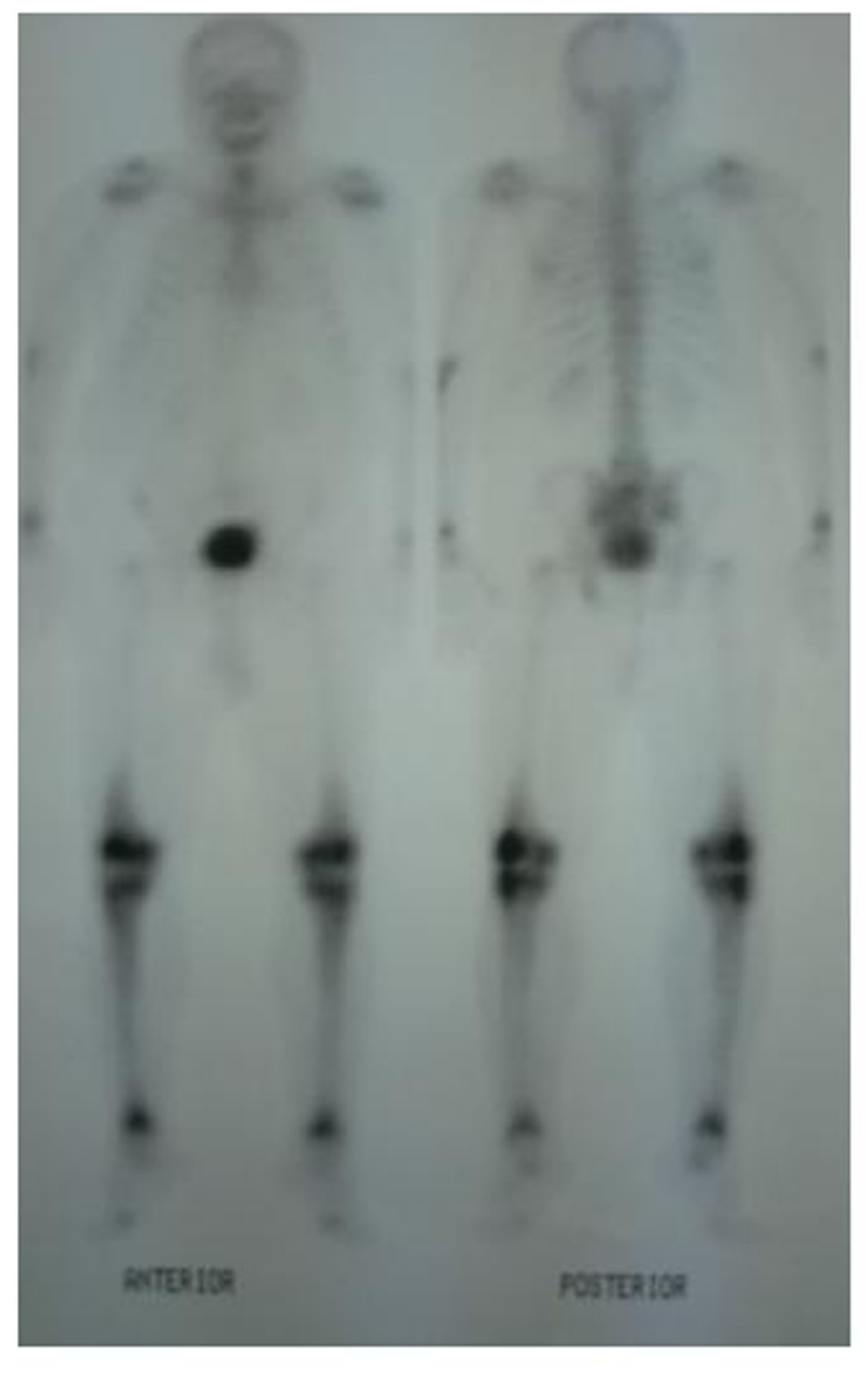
Increased osteoblastic activity was detected in bilateral proximal and distal tibia by whole-body bone scintigraphy.
